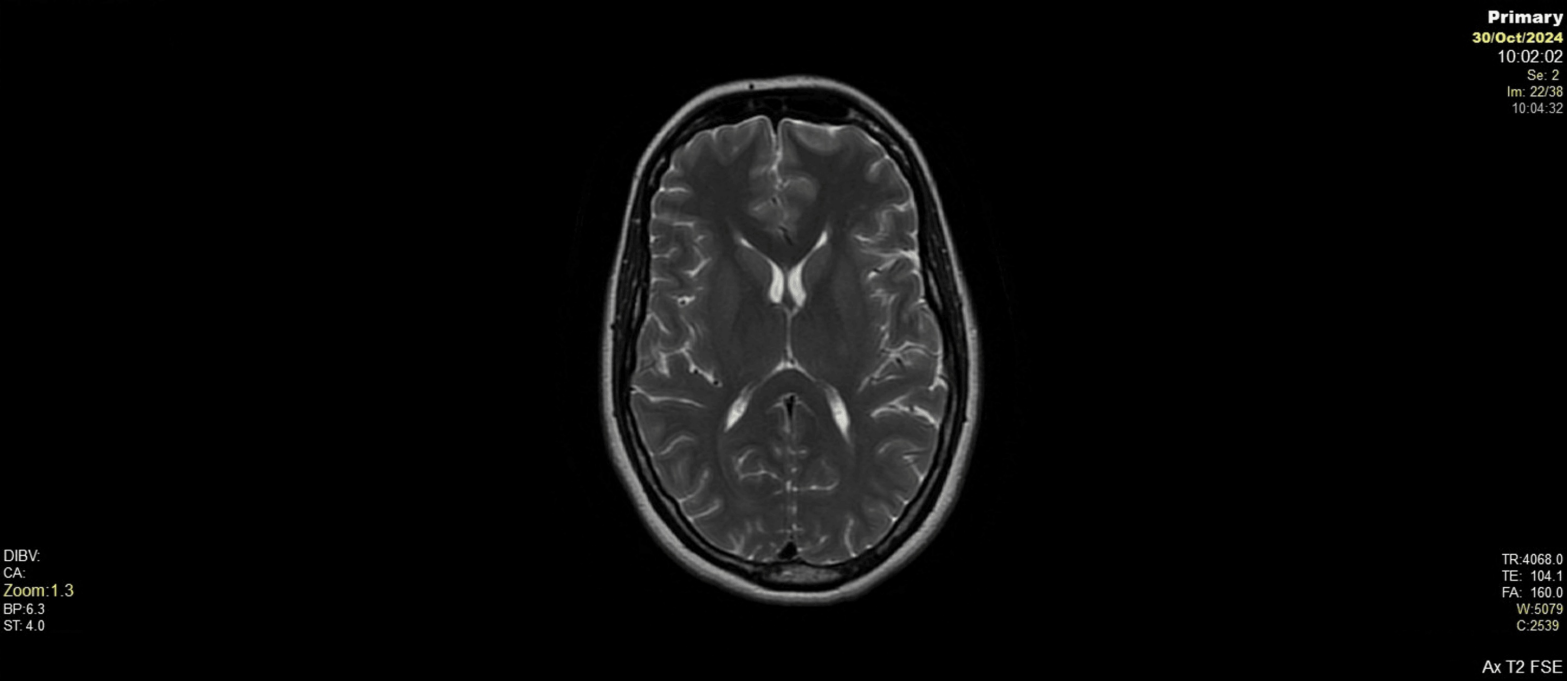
Axial T2-weighted MRI at lateral ventricles No evidence of cerebral oedema or abnormal signal intensity on T2-weighted axial images. The brain parenchyma appears normal with preserved grey-white matter differentiation. No signs of PRES or other postpartum-related intracranial abnormalities are identified.
